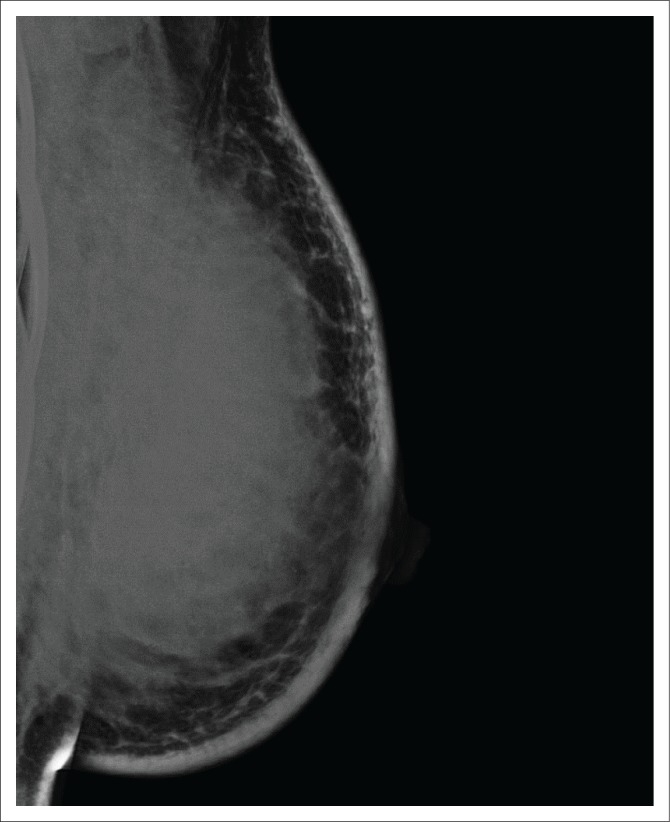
Mammogram (Left Medio-Lateral Oblique view) in a 39-year-old patient with a mass that fills the entire breast. There are inflammatory changes and extensive lymph node metastases. This patient was not a candidate for pre-treatment breast magnetic resonance imaging.
